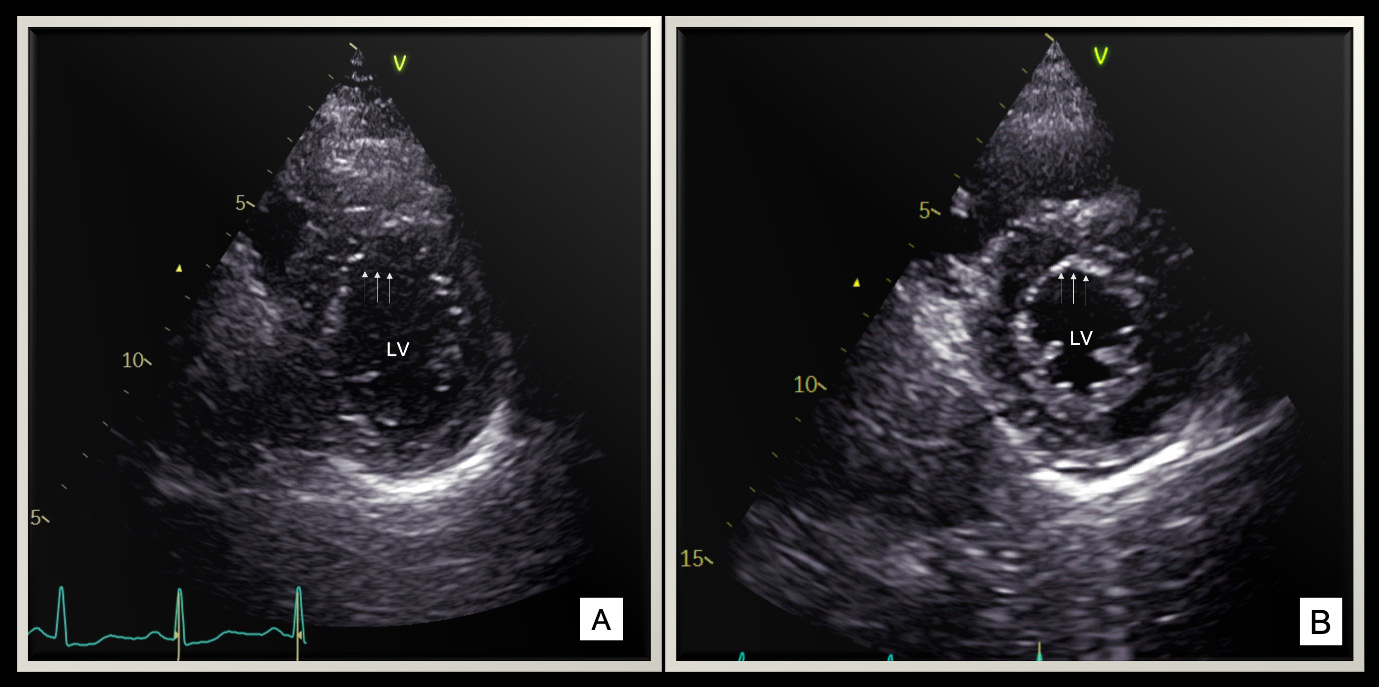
Table 2 T2: Echocardiographic characteristics of patients with Covid-19
